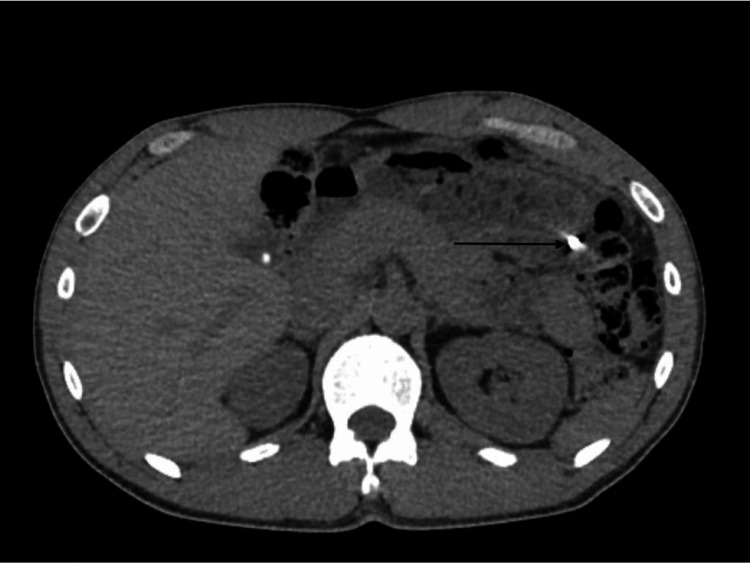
CT scan of FB ingestion (see arrow) Axial abdominal CT scan showing metallic artifact from FB in the distal gastric body. FB, foreign body; CT, computed tomography
